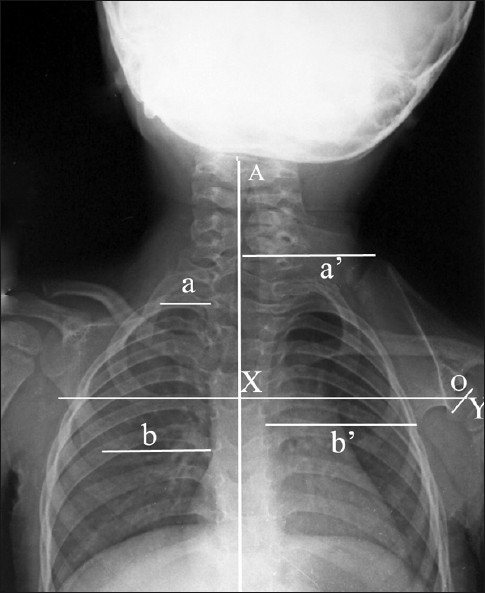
Frontal radiograph of a patient with Sprengel’s deformity (Rigault grade III). (X – Line drawn perpendicular to the body axis ‘A’; Y – Line joining the superior and inferior edges of the glenoid; O – Angle between lines X and Y; b: distance between the inferior angle of the normal scapula and the spine; b’ – Distance between the inferior angle of the affected scapula and the spine; a – The distance between the superior angle of the normal scapula and the spine; a’ – Distance between the superior angle of the affected scapula and the spine
